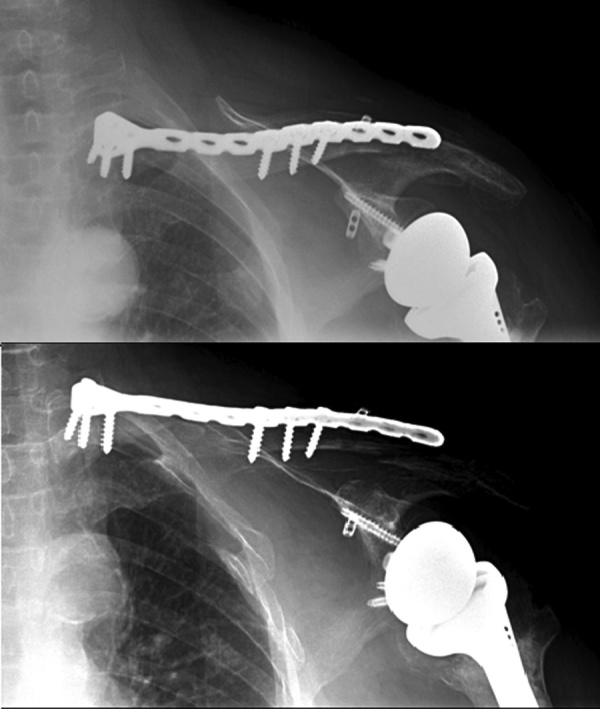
Radiograph of the left shoulder three months post op after removal of the percutaneous K-wires. Good bone healing seen with satisfactory alignment, with stable construct of the previous reverse shoulder prosthesis.
